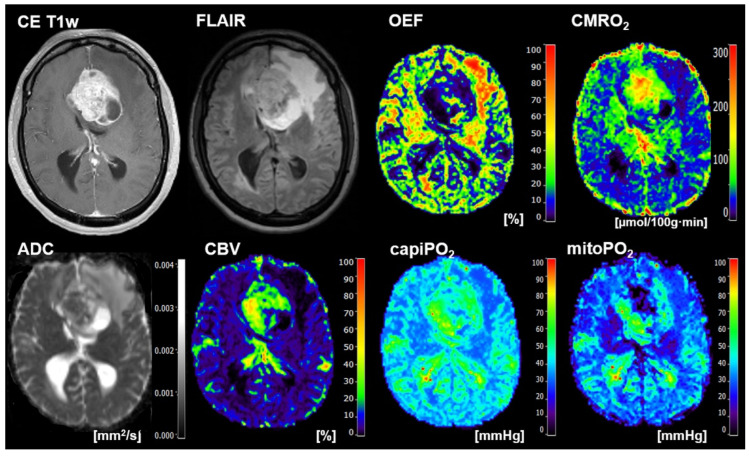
Representative case of a 47-year-old female patient suffering from a brain metastasis from breast cancer that was correctly classified by the 1D-CNN and CMRO_2_ features but misclassified as GB by the radiologists. Contrast-enhanced (CE) T1w and FLAIR MRI data combined with the quantitative maps of apparent diffusion coefficient (ADC) and cerebral blood volume (CBV) among others were used for human reading by two board-certified radiologists in consensus. MRI biomarker maps for oxygen metabolism including oxygen extraction fraction (OEF), the cerebral metabolic rate of oxygen (CMRO_2_), capillary oxygen tension (capiPO_2_), and mitochondrial oxygen tension (mitoPO_2_), respectively, of the patient are presented on the right-hand part of the Figure.
